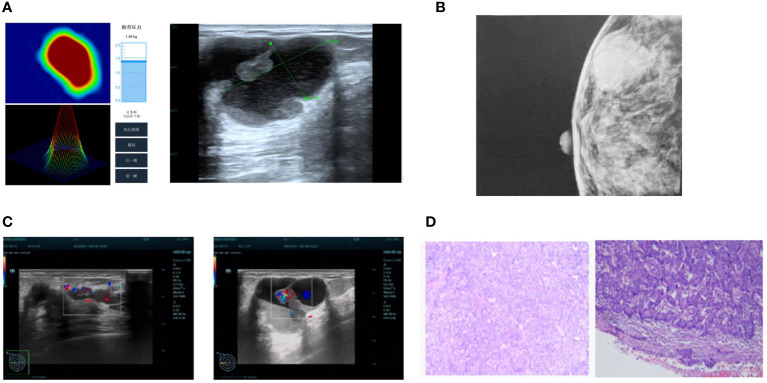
The visual difference between different methods for one patient. (A) Bimodal Breast Examination; (B) Mammography; (C) Ultrasound; (D) Pathology.
